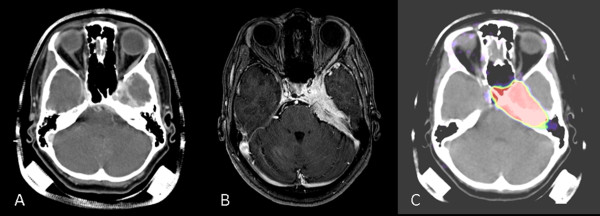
An example of the pretreatment pictures. (A) CT with contrast enhancement, (B) MRI with contrast enhancement and (C) 68 Ga-DOTATOC-PET for Case 2.
